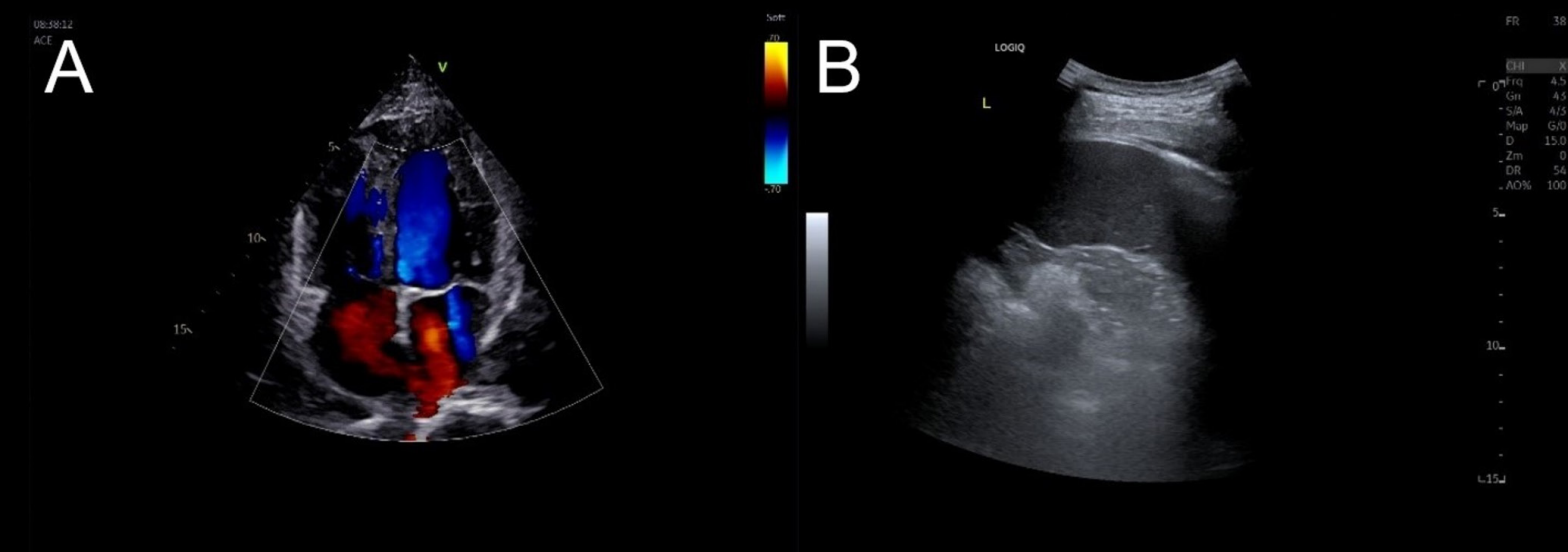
(A) Cardiac ultrasound did not show pericardial effusion; (B) thoracic ultrasound had no signs of pleural effusion.
